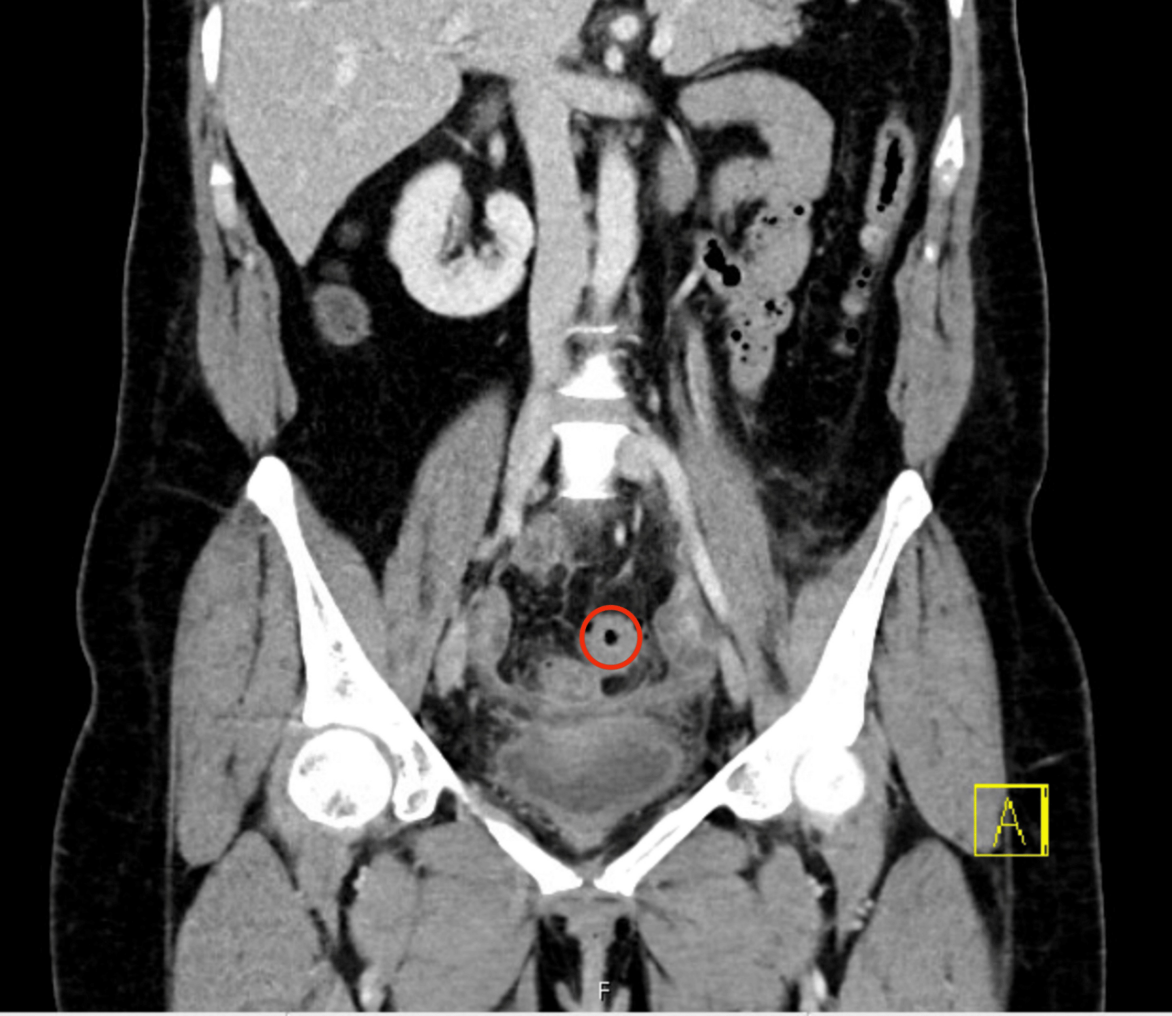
Transverse CT of the abdomen and pelvis demonstrating extraluminal air-fluid collection within the left pelvis
